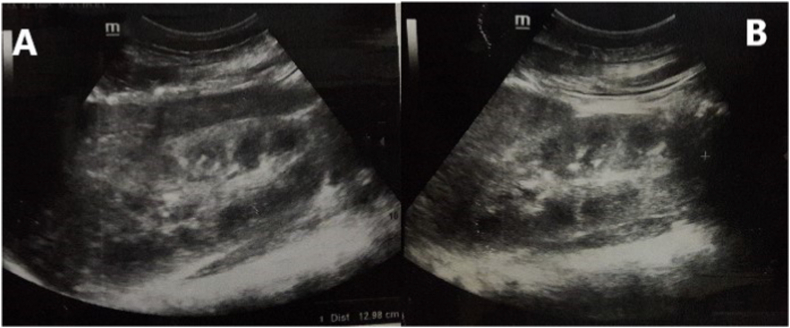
Renal ultrasound shows (A) right kidney diameter 12.9 cm, (B) lift kidney diameter 12 cm, and both kidneys show increase in cortical echogenicity, reduce corticomedullary differentiation and pyramids enlargement.
